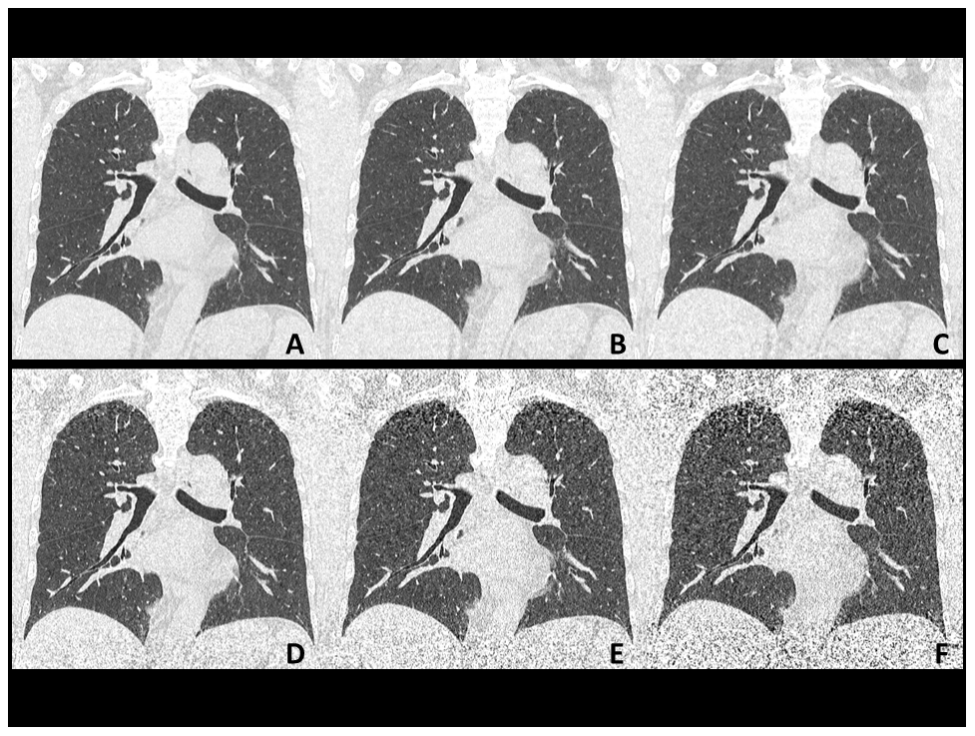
Reconstructed coronal plain chest CT images (56-year-old male weighing 62 kg). Images are arranged as in Figure 1 (A–C, with AIDR3D; D–F, without AIDR3D; A and D, at 240 mA; B and E, at 120 mA; C and F, at 60 mA). Severe image noise was observed at the upper lung zones and bottoms at 120 and 60 mA without AIDR3D (E and F), which was obviously improved using AIDR3D (B and C).
